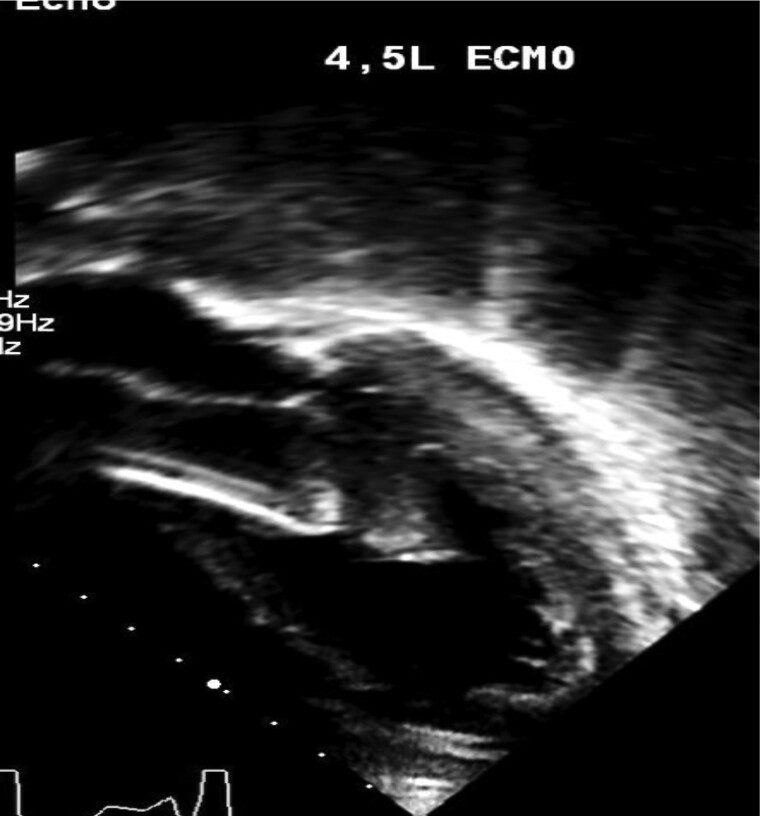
Still echocardiogram image showing Impella device in the left ventricle.
